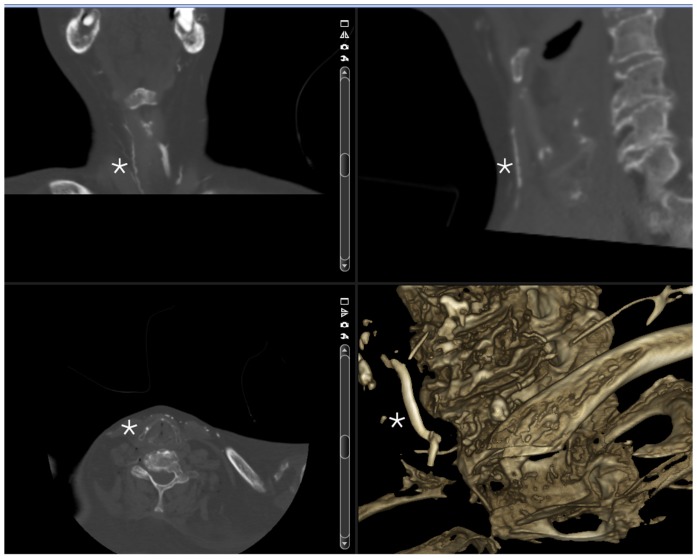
Multiplanar CT reconstruction and surface rendered image showing vessels (right to the asterisk) after barium sulfate injection into an intraosseous access placed into the left anterior mandible.
